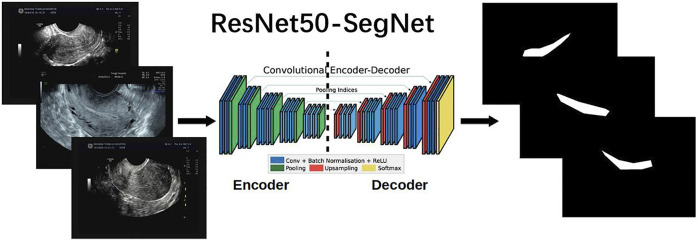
ResNet50-SegNet architecture [Modified from Badrinarayanan et al. (2015)] for semantic segmentation of endometrium from uterine ultrasound images.
